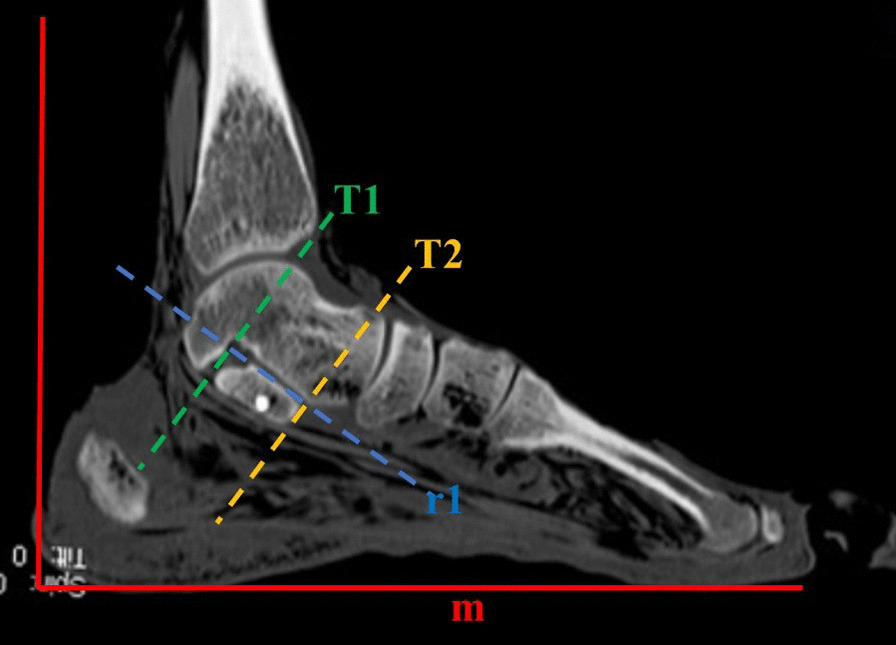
Sagittal CT image of the ankle joint. (r1) Upper surface of the sustentaculum tali; (T1) posterior edge of the sustentaculum tali; (T2) anterior edge of the sustentaculum tali
